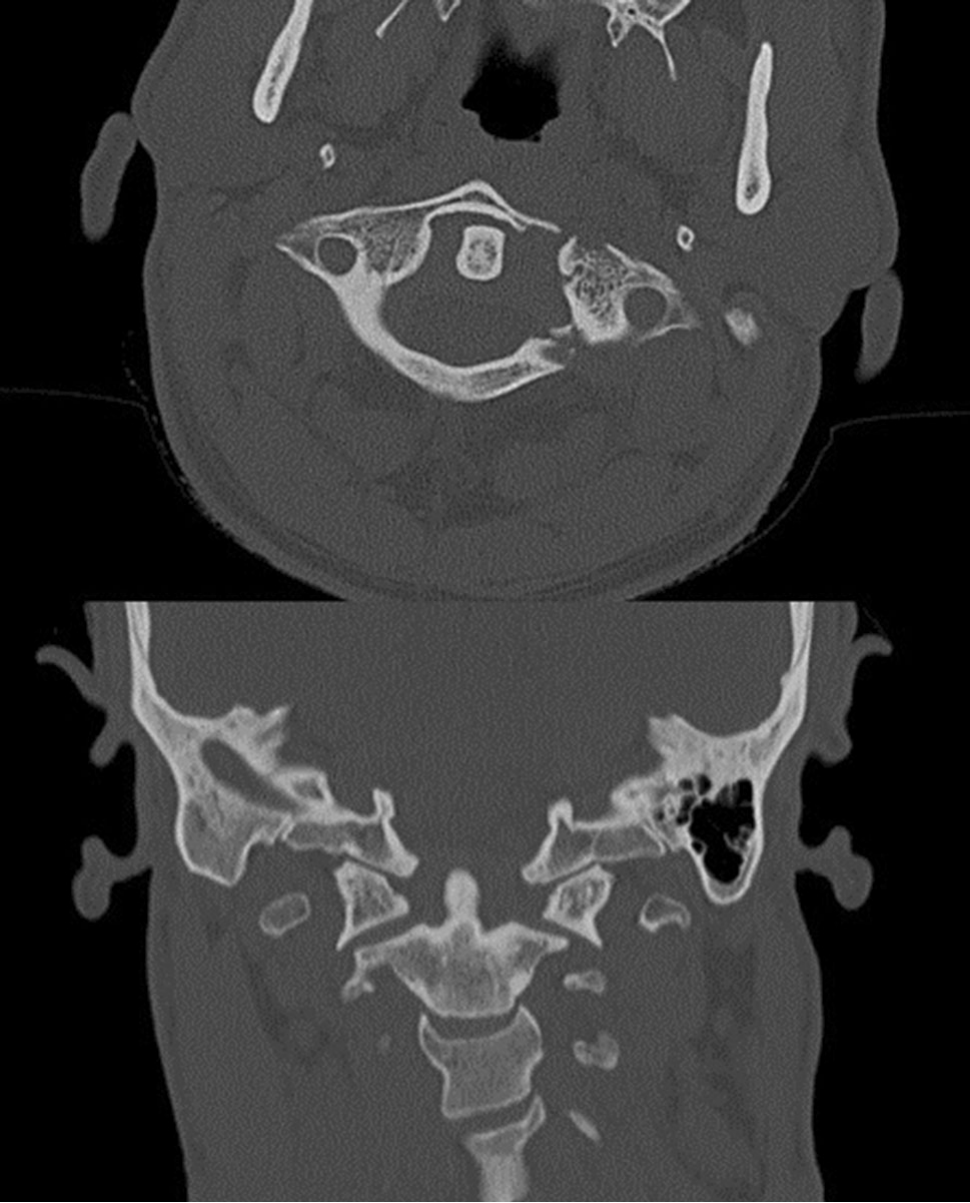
Transversal (*top*) and coronal (*bottom*) CT images of patient 2: Jefferson type III (Landells and Van Peteghem type II) fracture with dislocation of the left lateral mass of approximately 8 mm
